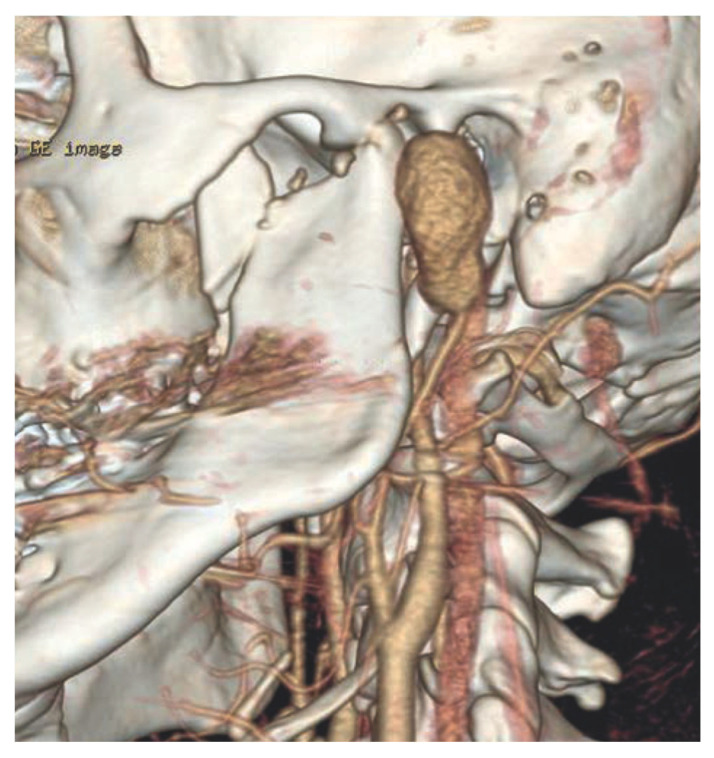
CT angiography. Visible pseudoaneurysm and fracture of the condylar head and coronoid process.
